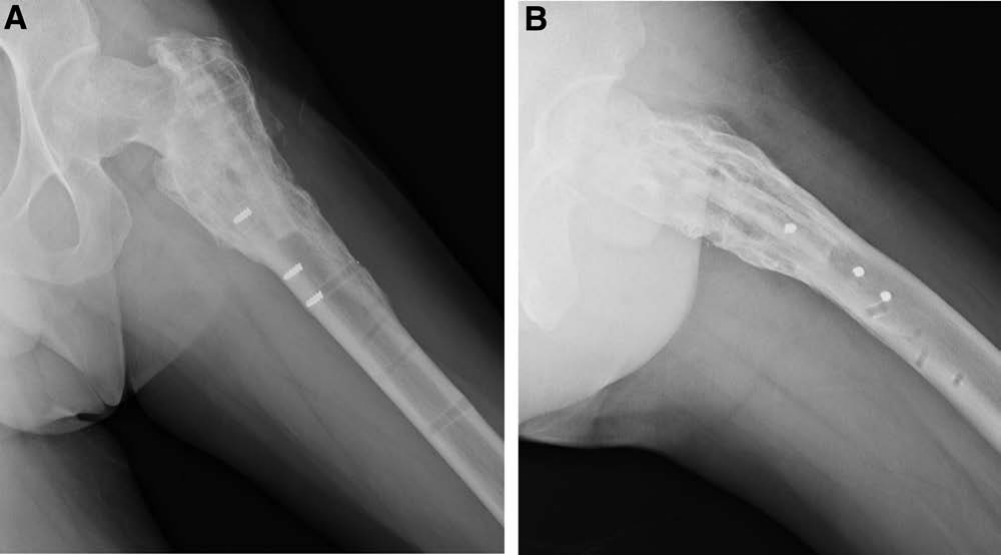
(A) Plain anteroposterior (AP) radiograph (B) lateral radiograph obtained after implant removal (3 years after fixation) display a union of intertrochanteric fracture.
